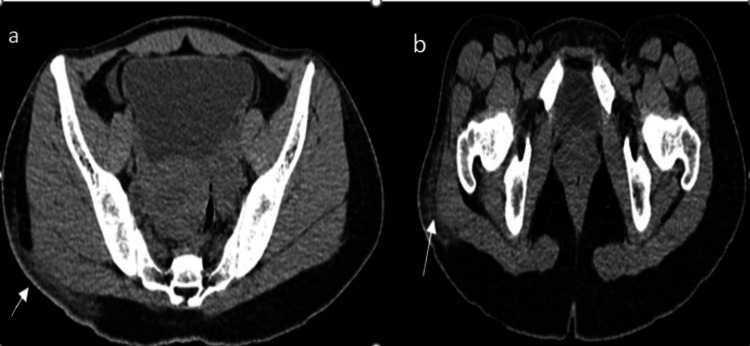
(a, b) CT showing a fairly defined area of fat stranding/inflammatory changes and relatively reduced subcutaneous fat in the posterolateral aspect of the right gluteal region.
